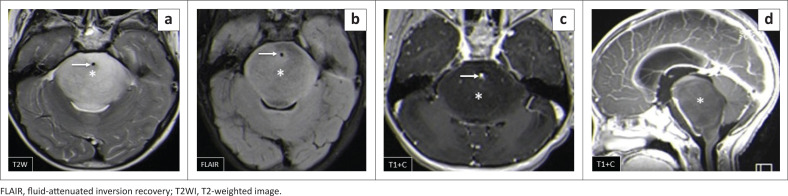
(a–d) Typical diffuse intrinsic pontine glioma that expands the pons (*) and encases the patent basilar artery (arrow). It is hypointense on T1WI, hyperintense on T2WI and FLAIR and does not enhance post-gadolinium. There was no restricted diffusion (not shown).
